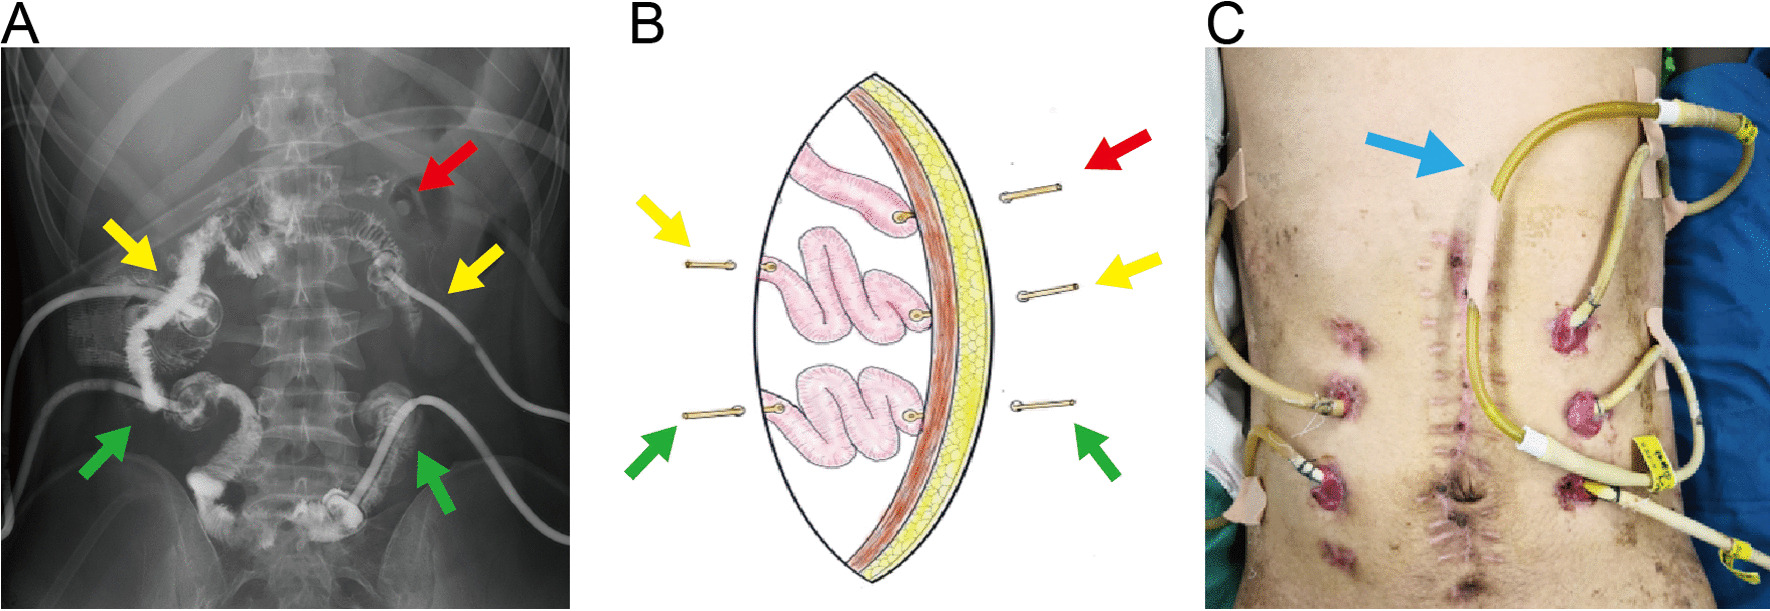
The CT image (A), graphical representation (B) explaining the location of the reserved small intestine marked as No. 1 (red arrows), No. 2 (yellow arrows) and No. 3 (green arrows). The photo (C) shows that the NO. 1 and NO. 2 intestines were bridged by rubber tubes(blue arrows). The intestinal movement direction was from left to right
